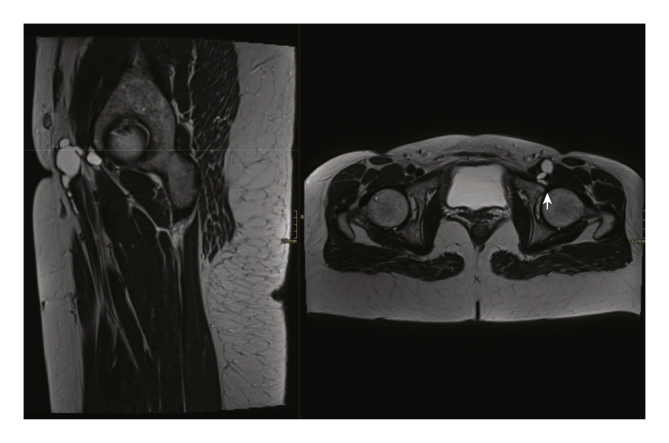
MRI images of the adventitial cyst in relation to the hip join on the left. T2 weighted images in the sagittal (left) and axial (right) plane showing the relationship between the cystic structure extending from the left hip joint (arrow) to the common femoral artery and proximal superficial femoral artery.
